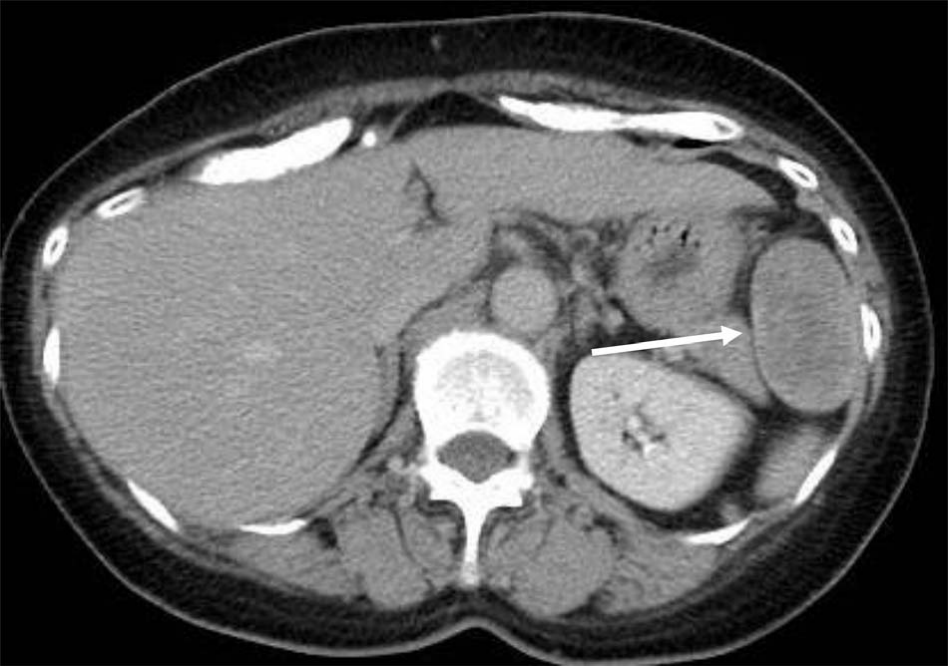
Radiological findings. Computed tomography showing a well-circumscribed soft tissue mass measuring 5.1 × 3.2 × 2.6 cm in the left anterolateral abdominal wall (white arrow).
